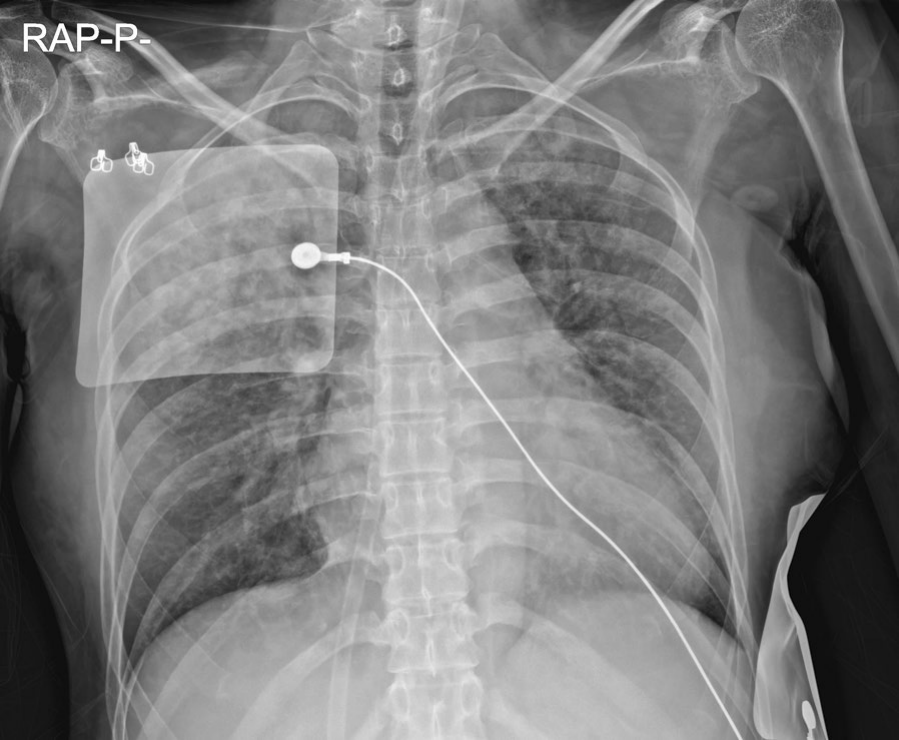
Initial chest X-ray reveals diffuse haziness with infiltration throughout the entire lung fields and prominent cardiomegaly
